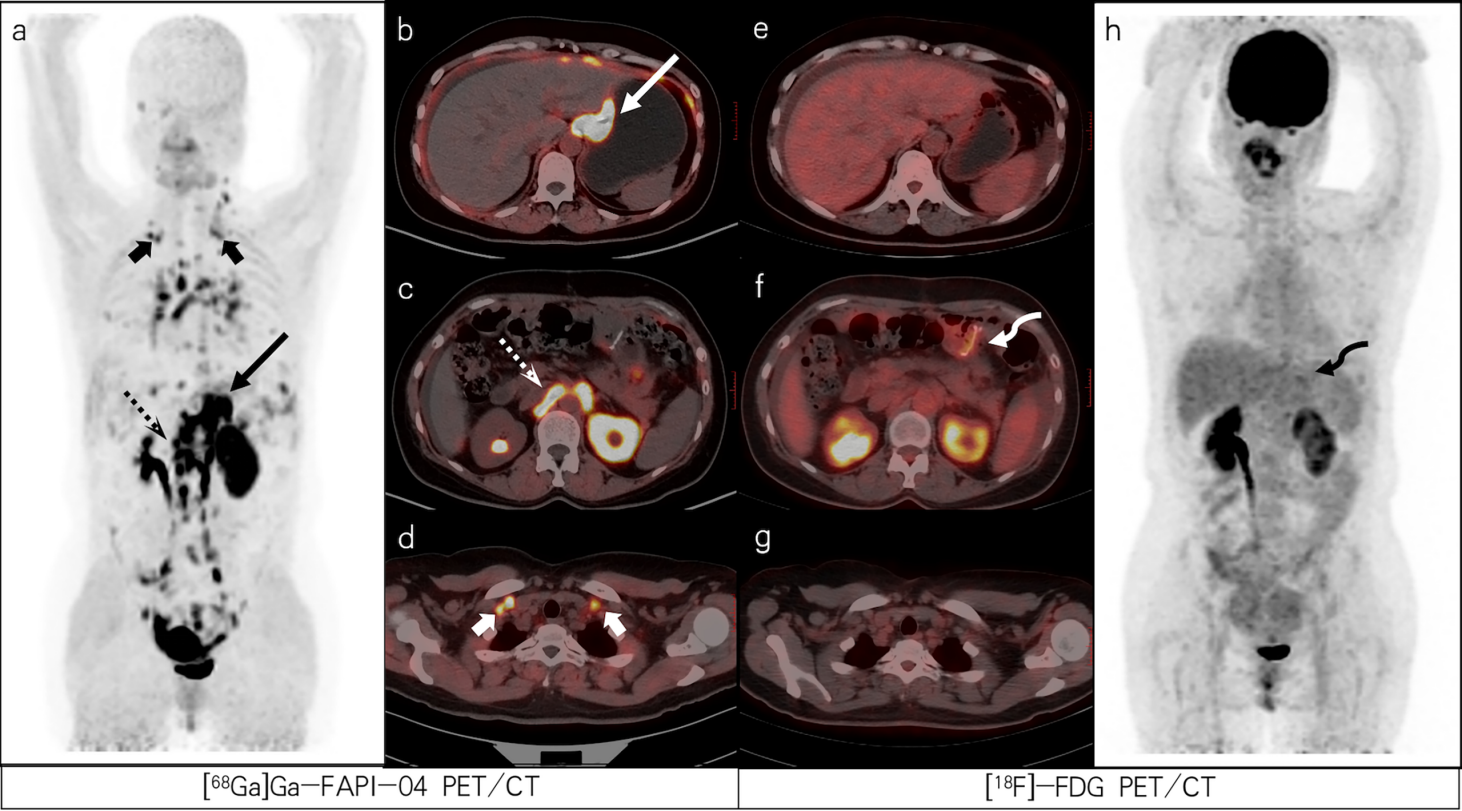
A 47-year-old female patient with poorly differentiated gastric adenocarcinoma with partial signet ring cells based on pathological biopsy after operation. [^68^Ga]Ga-FAPI-04 PET/CT (A–D) revealed high uptake in the gastric cardia (slender arrows, SUVmax=13.3), para-aortic lymph nodes (dashed arrows, SUVmax=15.7), and supraclavicular lymph nodes (short arrows, SUVmax=8.0) but negative uptake was observed on FDG PET/CT (E–H). [^18^F]-FDG PET/CT (F) revealed increased uptake in the gastric anastomosis (bent arrow, SUVmax=3.2) but negative uptake was observed on [^68^Ga]Ga-FAPI-04 PET/CT (C). The lesion was ultimately confirmed as residual gastritis by gastroscopic biopsy.
